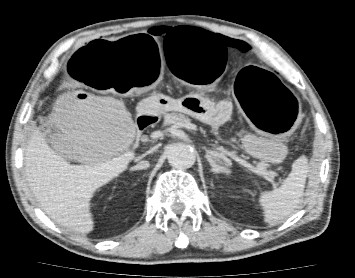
Abdominal computed tomography on the fifth postoperative day. A CT scan revealed severe colonic dilation. The diameter of the transverse colon was over 6cm. However, colonic wall thickening was absent.
